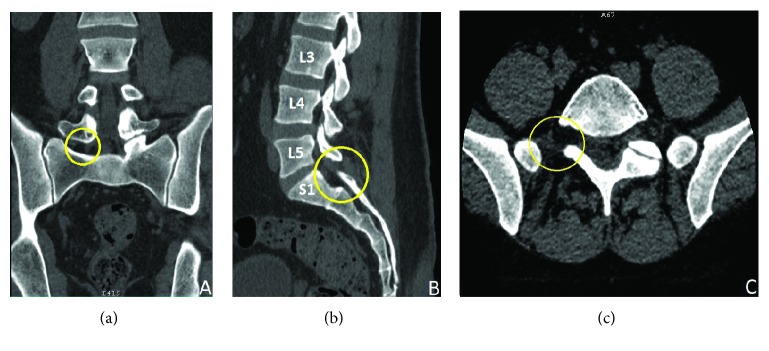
(a) Coronal cut of spine CT scan showing the absence of the right L5-S1 zygapophyseal joint forming a defect. (b) Sagittal cut of spine CT scan showing the absence of the L5 inferior articular process on the right with normal neighboring joints. (c) Axial CT scan cut of the spine further confirming the absence of the L5-S1 facet joint on the right side.
